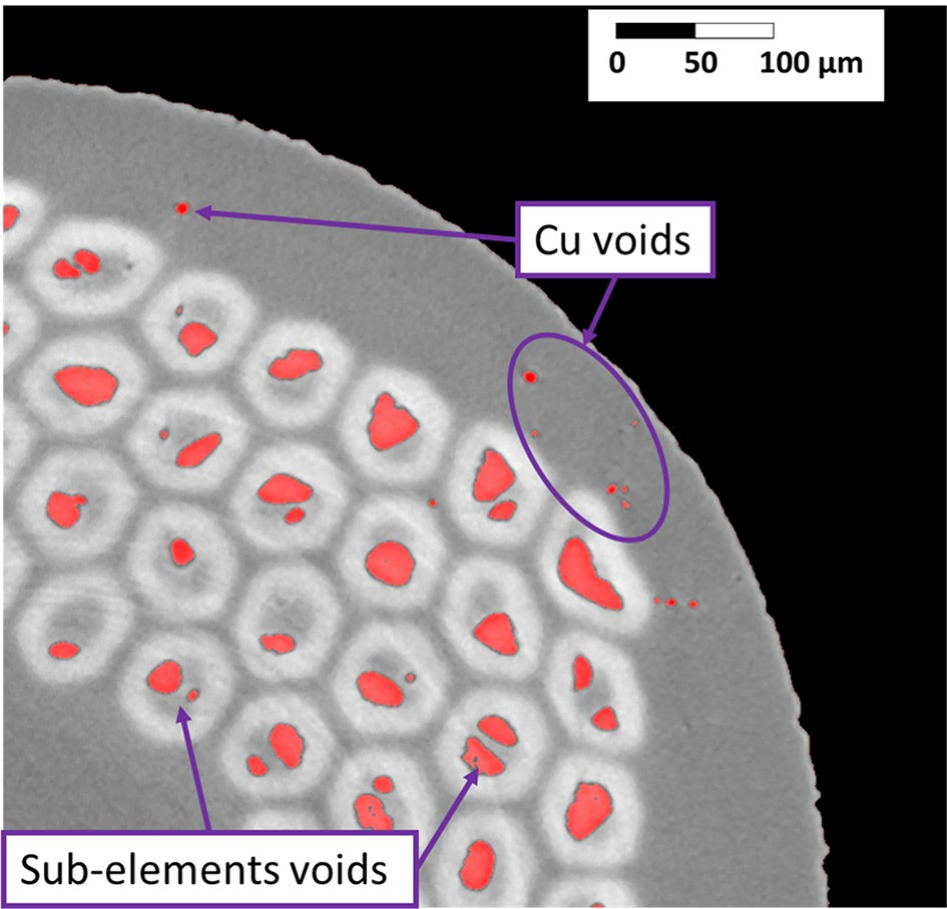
Detection of the voids in a x-ray tomography of the sample S20. Cu and sub-elements voids are in red.
